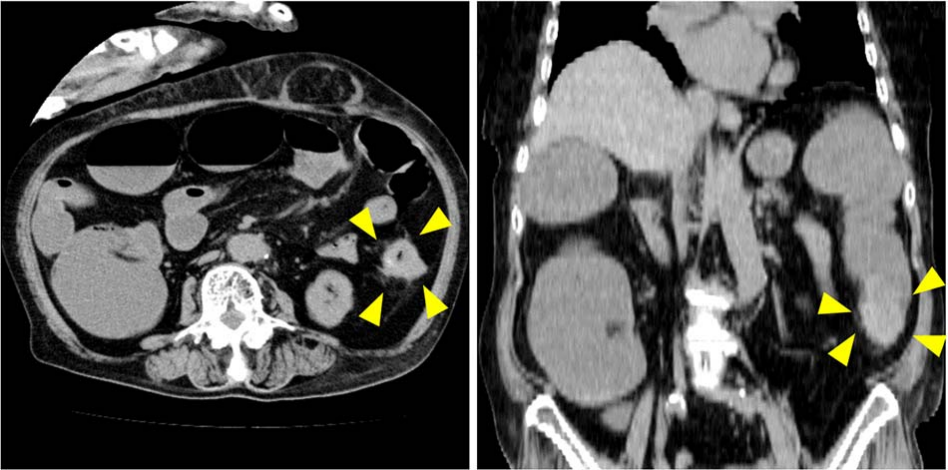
Computed tomography on arrival at our hospital revealed irregular wall thickening in the descending colon (arrow), accompanied by intestinal dilation proximal to the affected area. Obstructive ileus caused by cancer of the descending colon was suspected.
